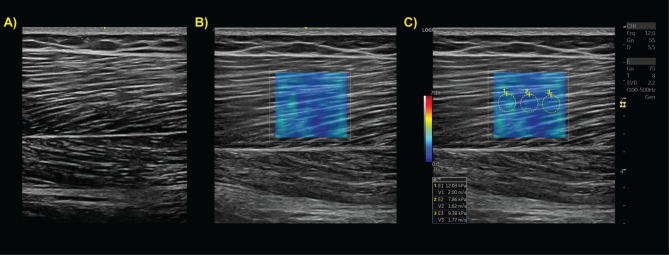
Representative ultrasound (A) B-mode image of the mid-belly biceps femoris long head muscle, (B) shear wave map at the same location, and (C) Q-box measures within the shear wave map with corresponding values in bottom lefthand subpanel
